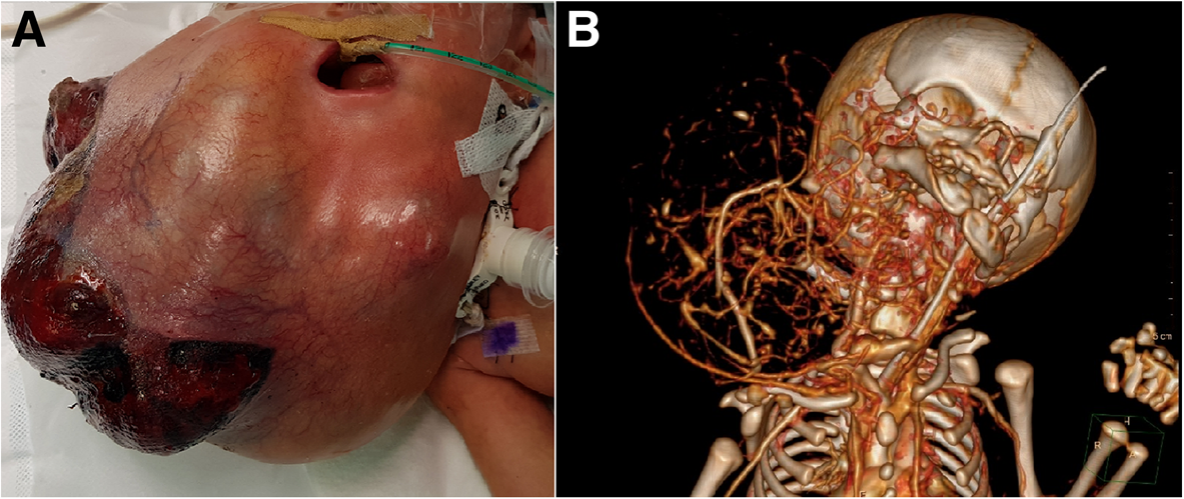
a The giant congenital teratoma on the third day of life. The tumor was covered with a thin skin layer with a large cystic and solid appearance. There were occasional bleedings from several lacerated areas on the tumor surface. b A computed tomography study demonstrated the abundant blood supply of the tumor including the fact that the right carotid artery was supplying this tumor
